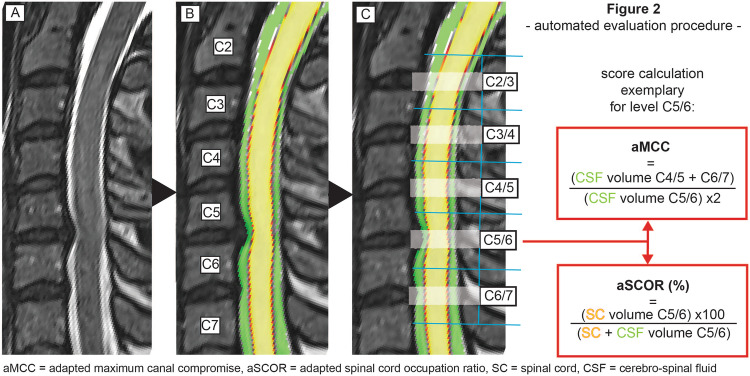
Overview of the fully-automated evaluation procedure of an exemplary patient with cervical stenosis in C5/6. A High-resolution 3D T2-weighted images. B Segmentation of spinal cord (yellow) and CSF space (green) with determination of the vertebral bodies from C2 to C7. C Calculation of CSF and spinal cord volumes at the middle third of each evaluated segment (white shaded rectangle). Right: Formula for aMCC (CSF space proportion of the index to both surrounding segments) and aSCOR (proportion of spinal cord and CSF space at the index segment) as objective parameters.
